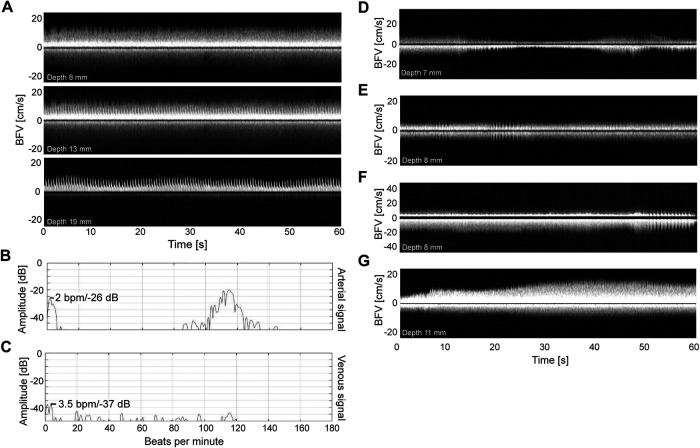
Examples of venous signals and the relationship between arterial and venous signals. (A) the Doppler velocity curves from a vein at 8 mm depth (upper panel), an artery at 19 mm depth (lower panel), and their relation to each other at 13 mm depth (middle panel). (B,C) the frequency spectrums of the vein (8 mm) and artery (19 mm) with beats/min along the *x*-axis and amplitude (decibel) along the *y*-axis. (D–G) Five typical examples of venous velocity measures. Each recording is from different neonatal subjects. Example 3D,E is recorded during sleep and 3F,G is recorded during wakefulness. The *x*-axis represents time of recording (seconds) and the *y*-axis represents velocity (cm/second).
